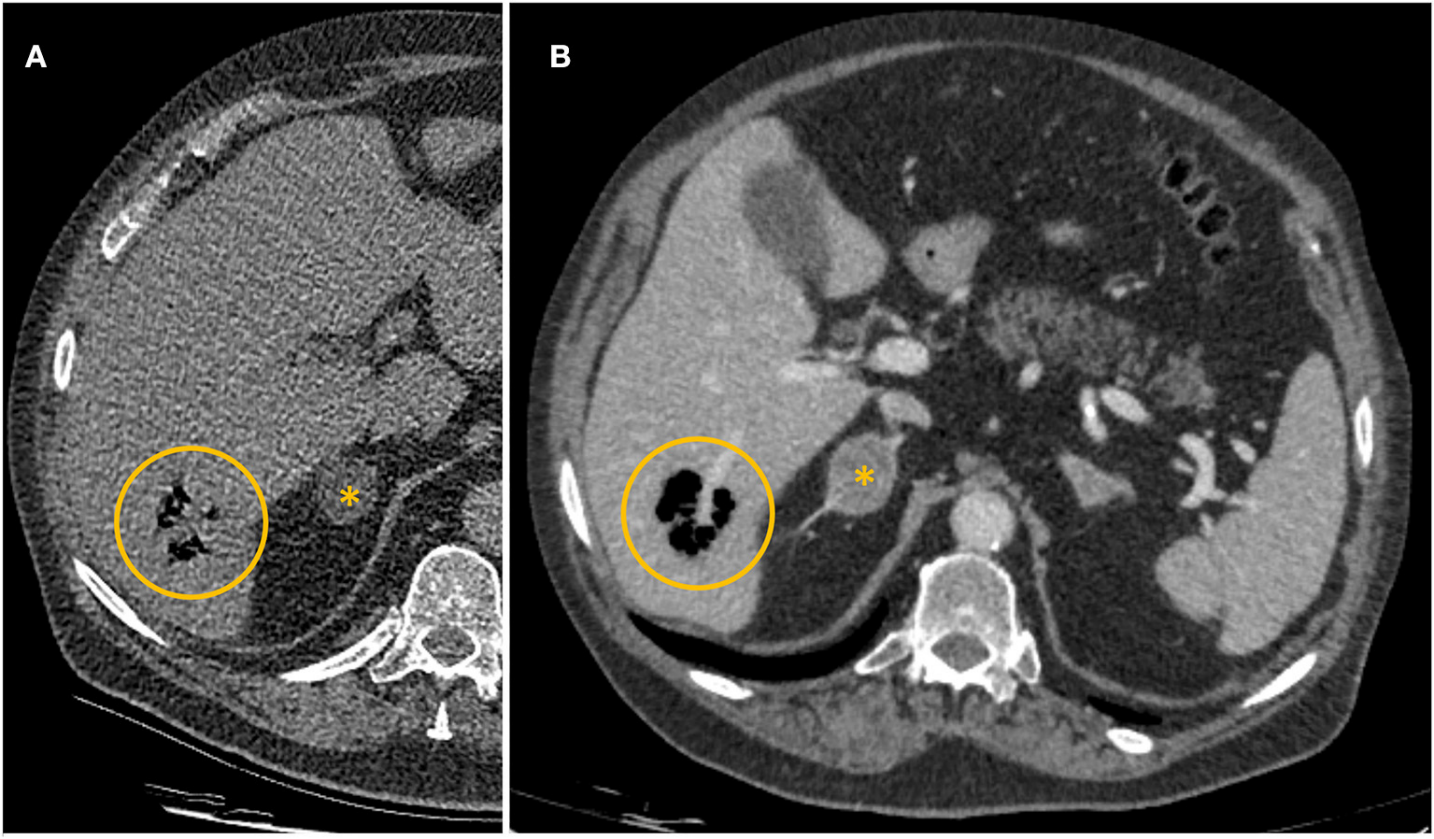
(A) Axial computed tomography pulmonary angiogram on admission. Collection of gas in liver segment 6 (yellow circle). (B) Contrast-enhanced computed tomography of the abdomen 154 min after the first CT scan. Collection of gas in liver segment 6 (yellow circle). Incidental finding of a mass of the right adrenal gland (^*^).
